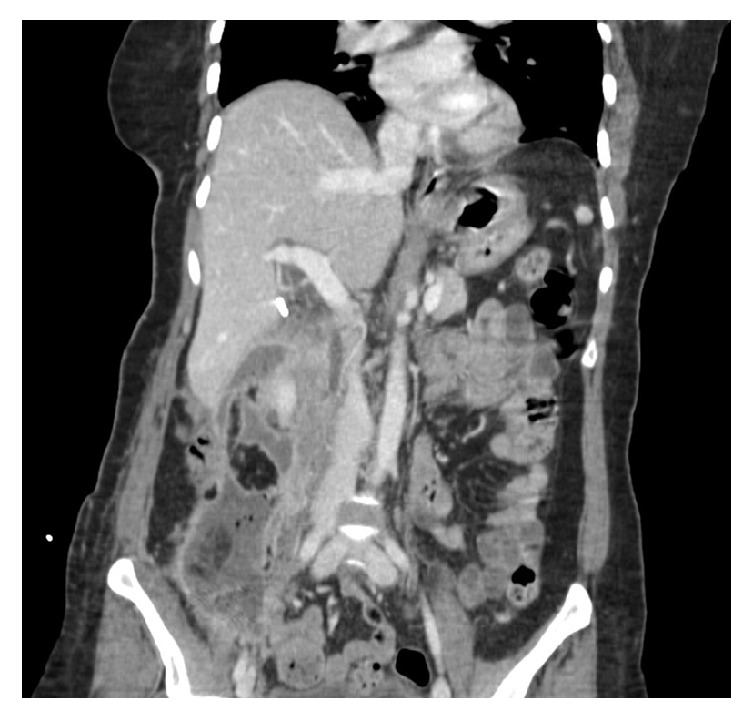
Coronal abdominal CT scan image showing retroperitoneal abscess.
